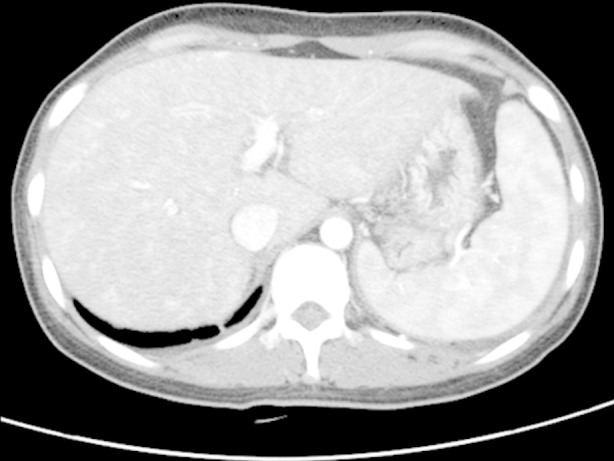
Scrub typhus in a 43-year-old woman. Arterial phase dynamic CT images show mild inhomogeneous enhancement of the hepatic parenchyma as well as splenomegaly.
